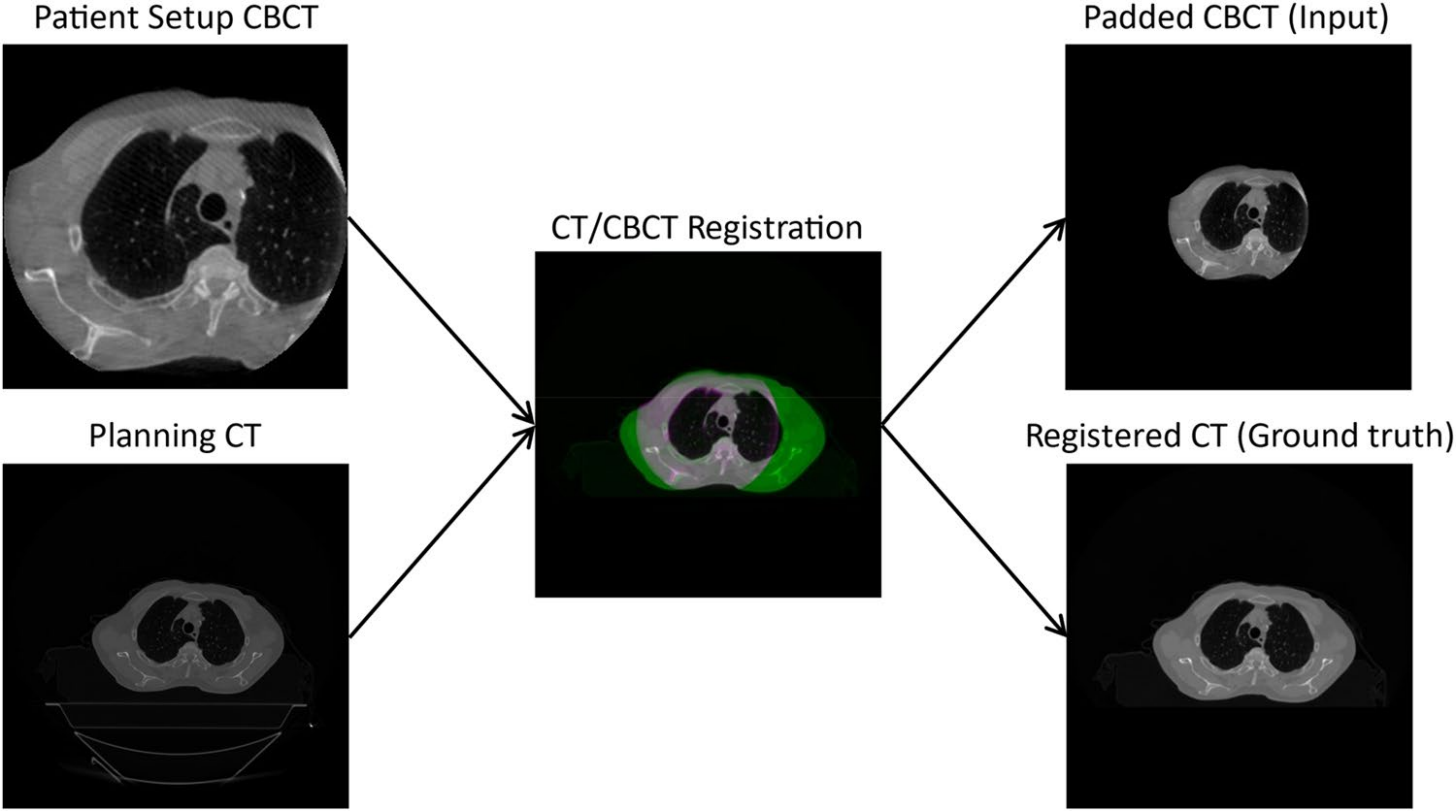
Creation of the padded CBCT and registered CT for input to the base clinical model
